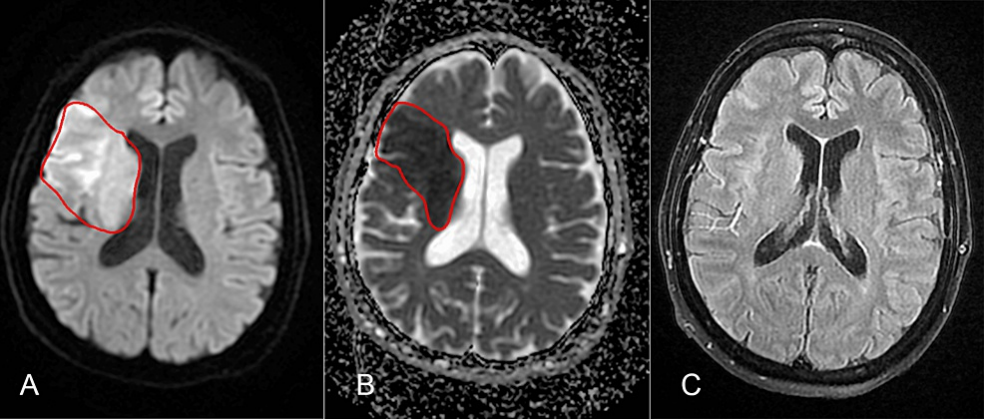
Cerebral MRI performed shortly after the stroke onset. (A) Diffusion-weighted imaging sequence; (B) apparent diffusion coefficient sequence; (C) fluid-attenuated inversion recovery sequence reveals a recent ischemic stroke in the territory of the right middle cerebral artery.
